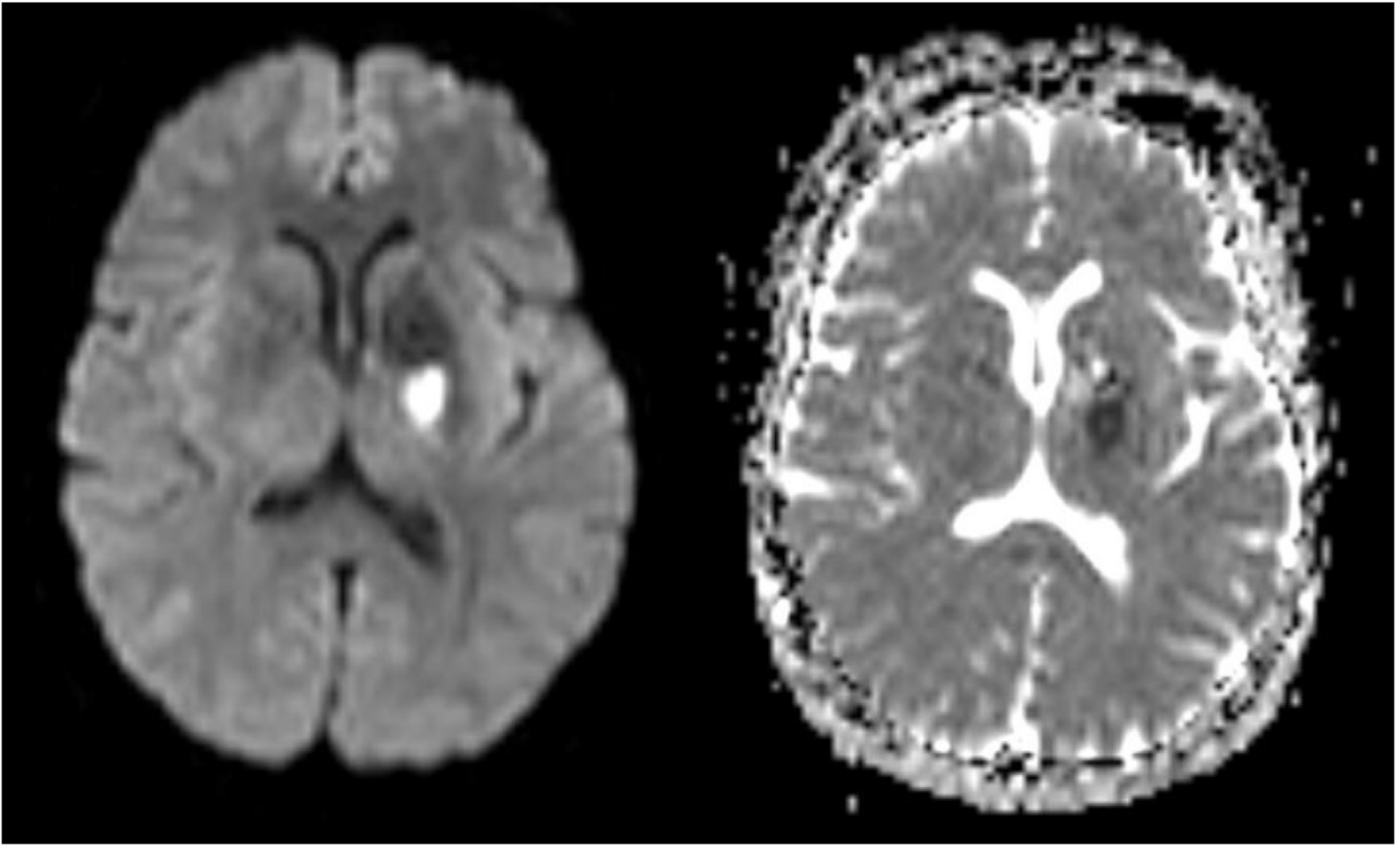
Diffusion-weighted imaging of the brain (left) and corresponding apparent diffusion coefficient (ADC) map (right) demonstrates an acute left gangliocapsular infarct diagnosed in 2011. The patient’s presenting complaint of acute-onset right lower extremity weakness was concordant with MRI findings.
